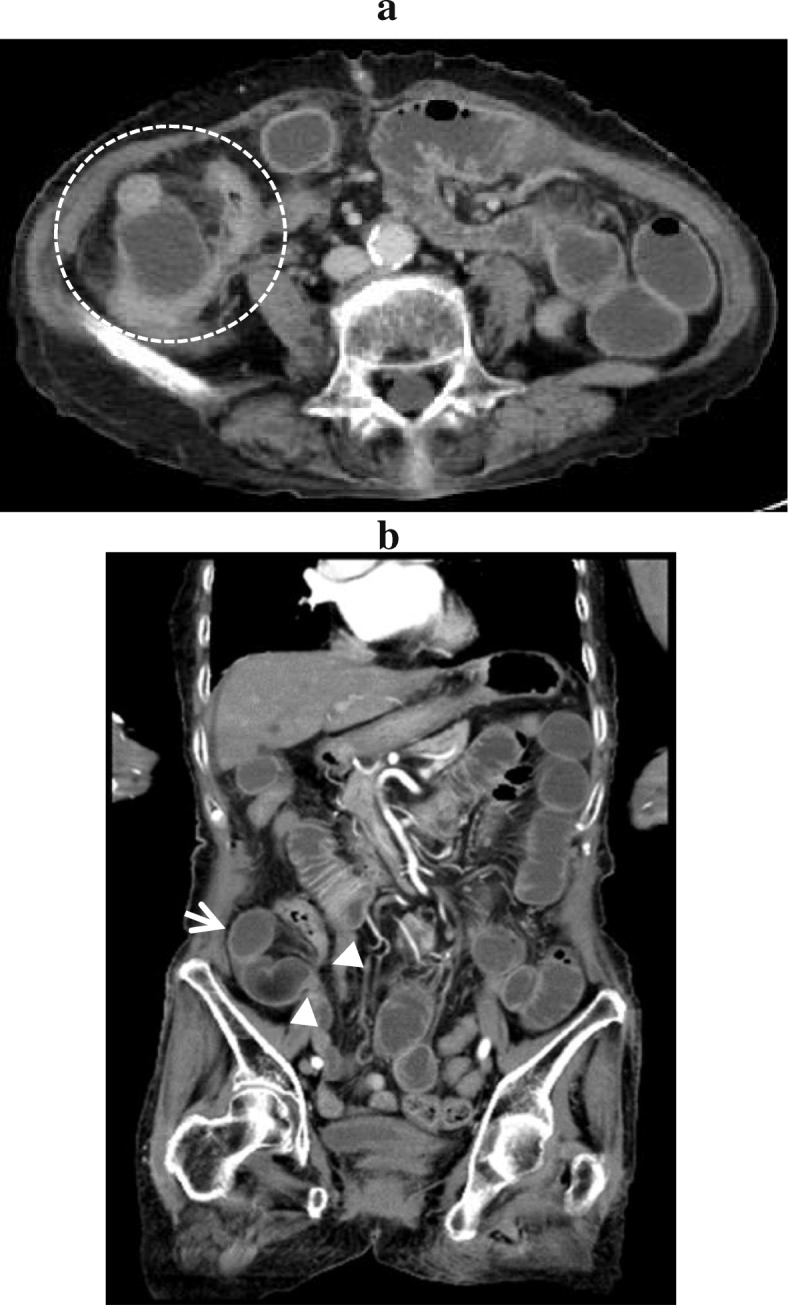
Abdominal enhanced computed tomography (CT) scan on admission. a Axial CT scan shows the small intestine in the right paracolic gutter and medially displaced ascending colon. b Coronal CT scan shows the closed loop sign of the small intestine in the right paracolic gutter (arrow) and hernia orifice (arrow head)
